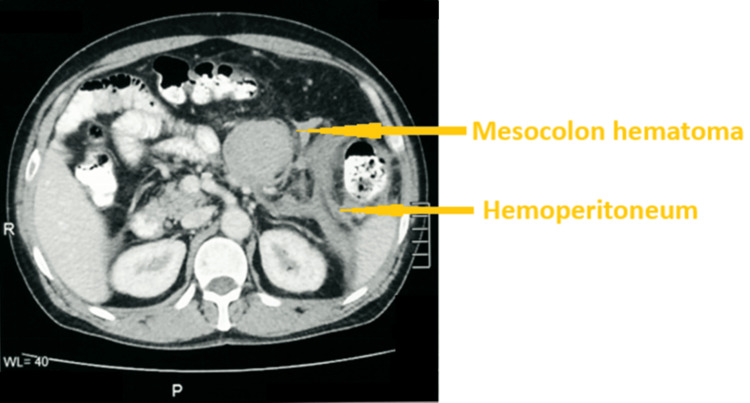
Axial CT image showing mesocolon hematoma with hemoperitoneum
